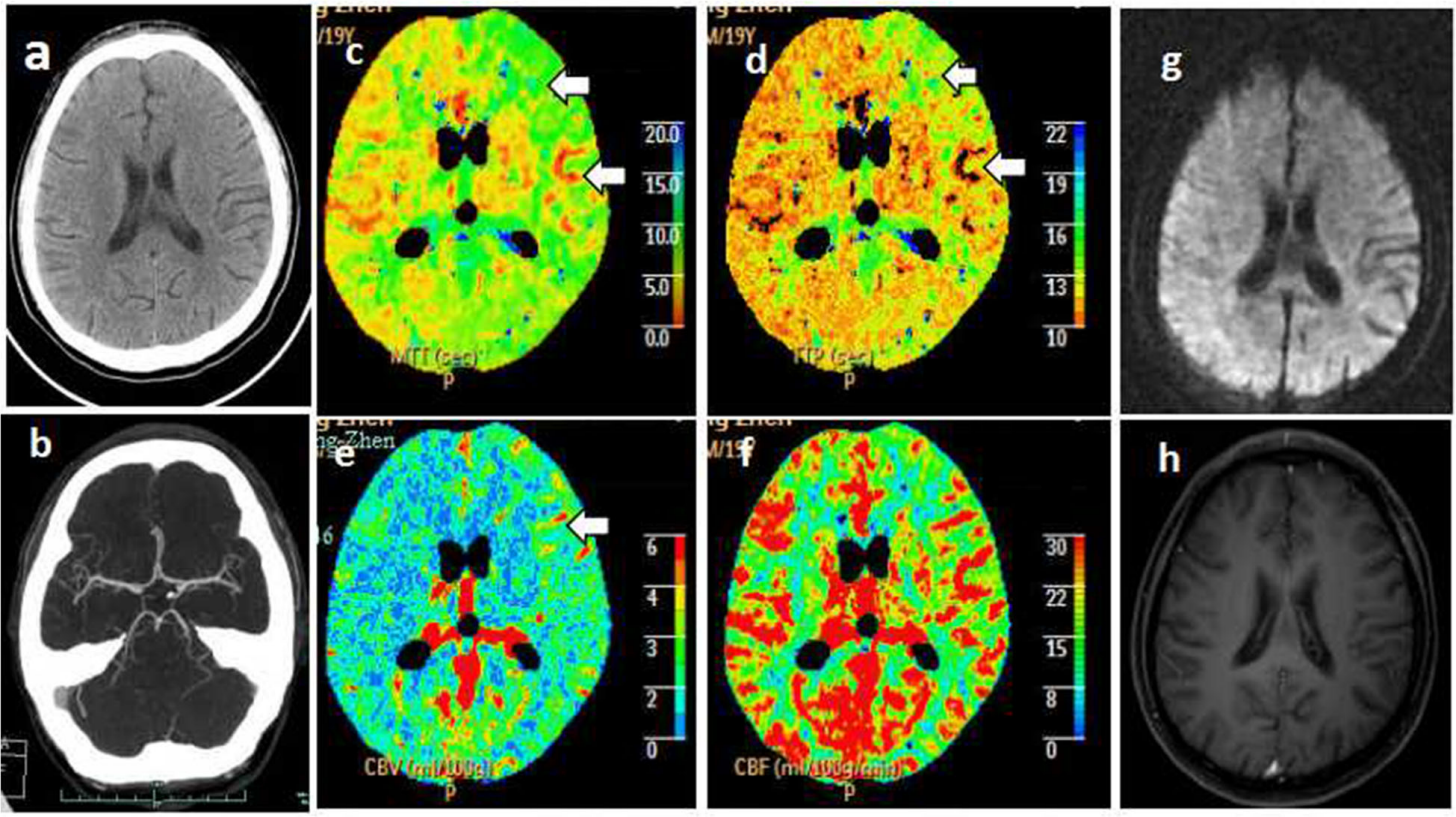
The patient’s neurologic images. a–f Mutimodal CT obtained 2 h after onset: (a) Normal nonenhanced CT. b Normal intracranial CTA. c–f Color maps of CTP showed prolonged MTT (c) and TTP (d) in the left hemisphere. The corresponding CBV (e) was slightly increased, and the CBF (f) was relatively preserved. g DWI of brain MRI obtained the next day was normal. h Brain contrast-enhanced MRI showed no meninges or brain parenchymal enhancement
